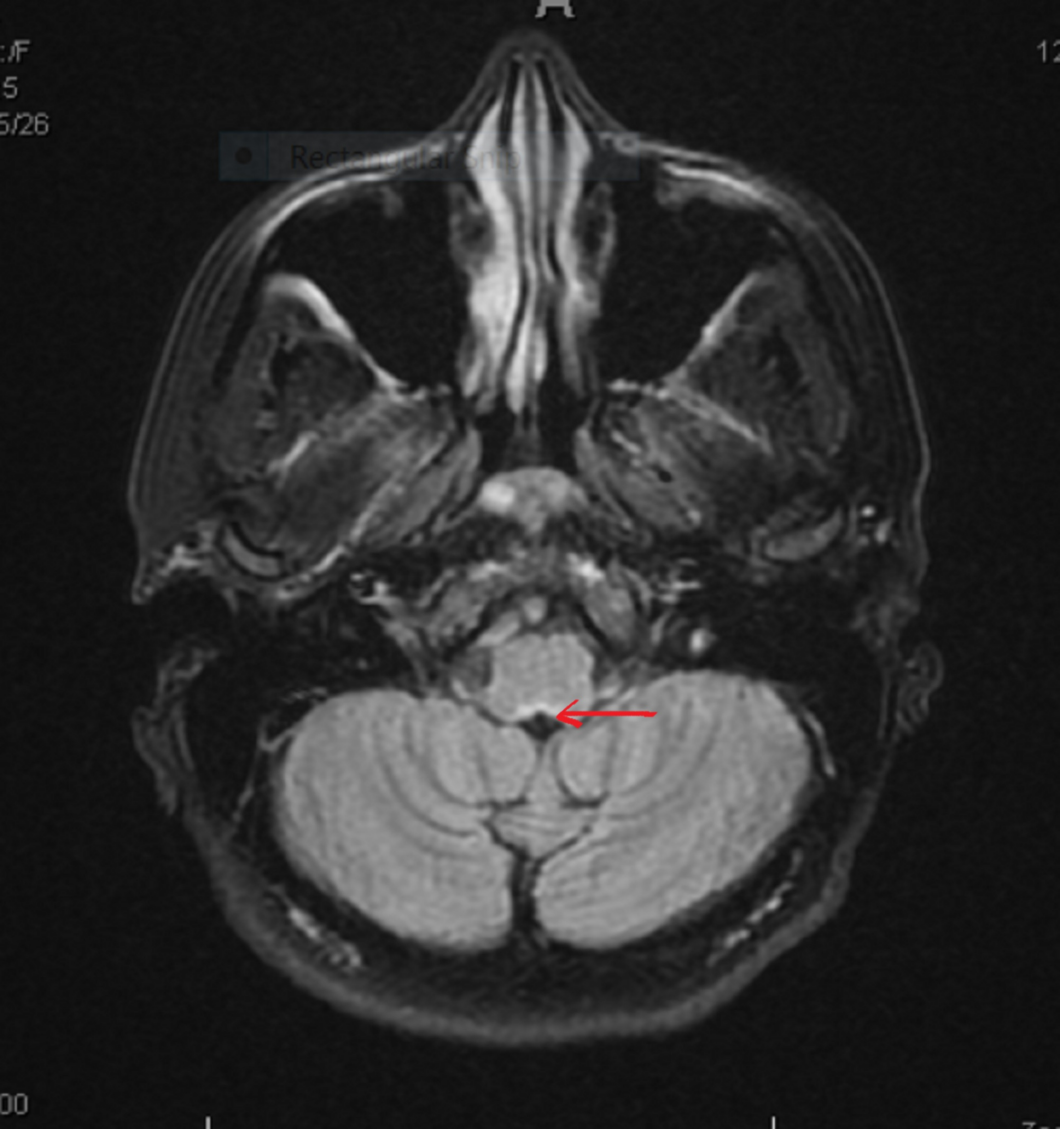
Repeat MRI brain coronal section showed only old lesion at area postrema, no new lesions (arrow pointing).
